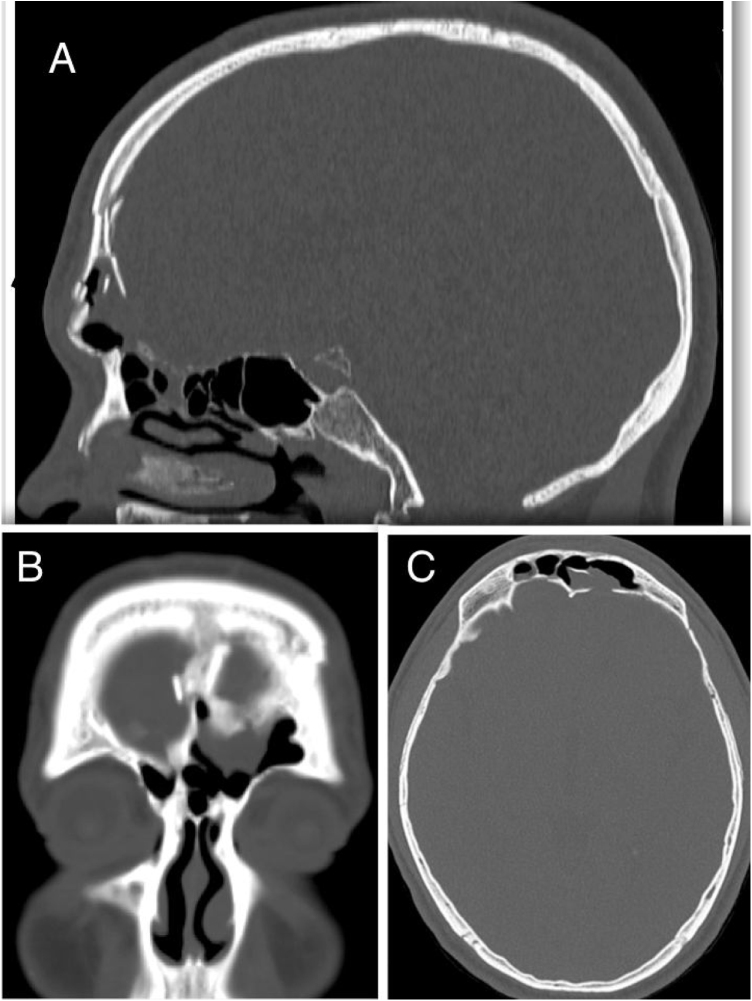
Triplanar CT scan (A, sagittal; B, coronal; C, axial planes) of a patient with traumatic CSF leak. Multiple skull base defects (frontal sinus posterior wall and ethmoid roof) are associated with anterior sinus wall fracture.
